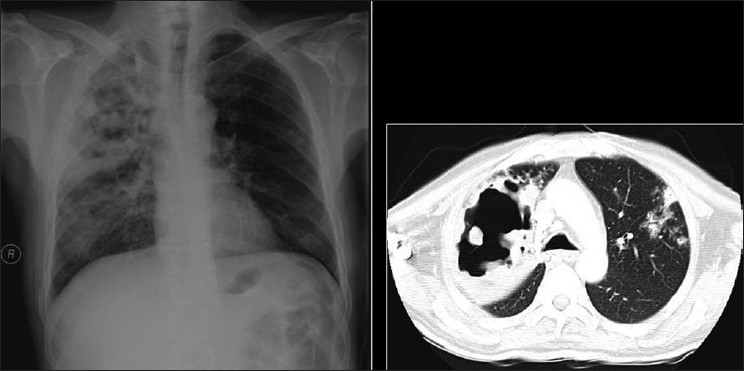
Necrotizing cavitating pneumonia. Chest X-ray and computed tomography depicting necrotizing cavitating pneumonia due to *Staphylococcus aureus* in a 29-year-old male with acquired immunodeficiency syndrome
